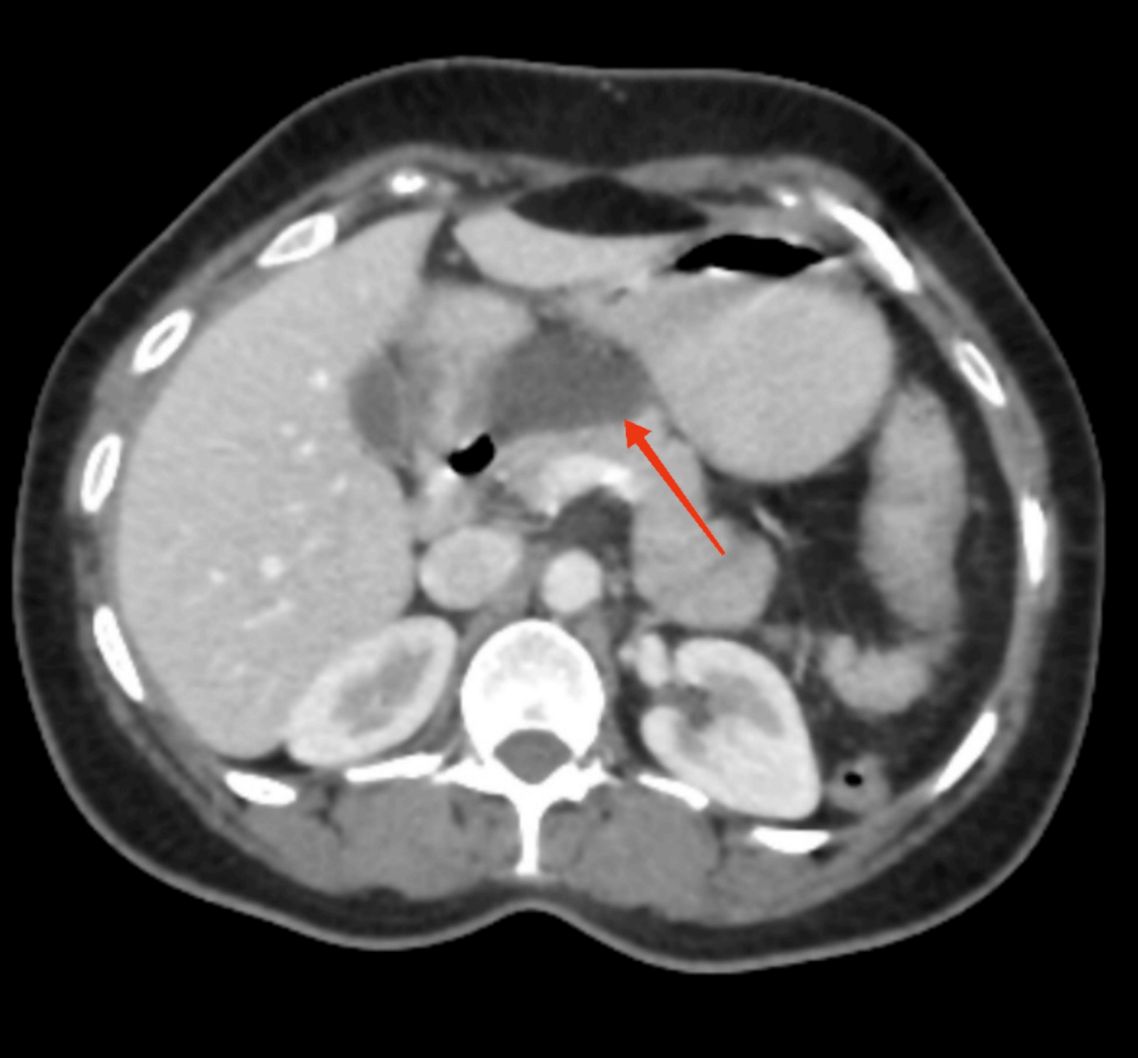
Computed tomography scan in coronal view showing the peripancreatic cyst The red arrow shows the peripancreatic cyst
